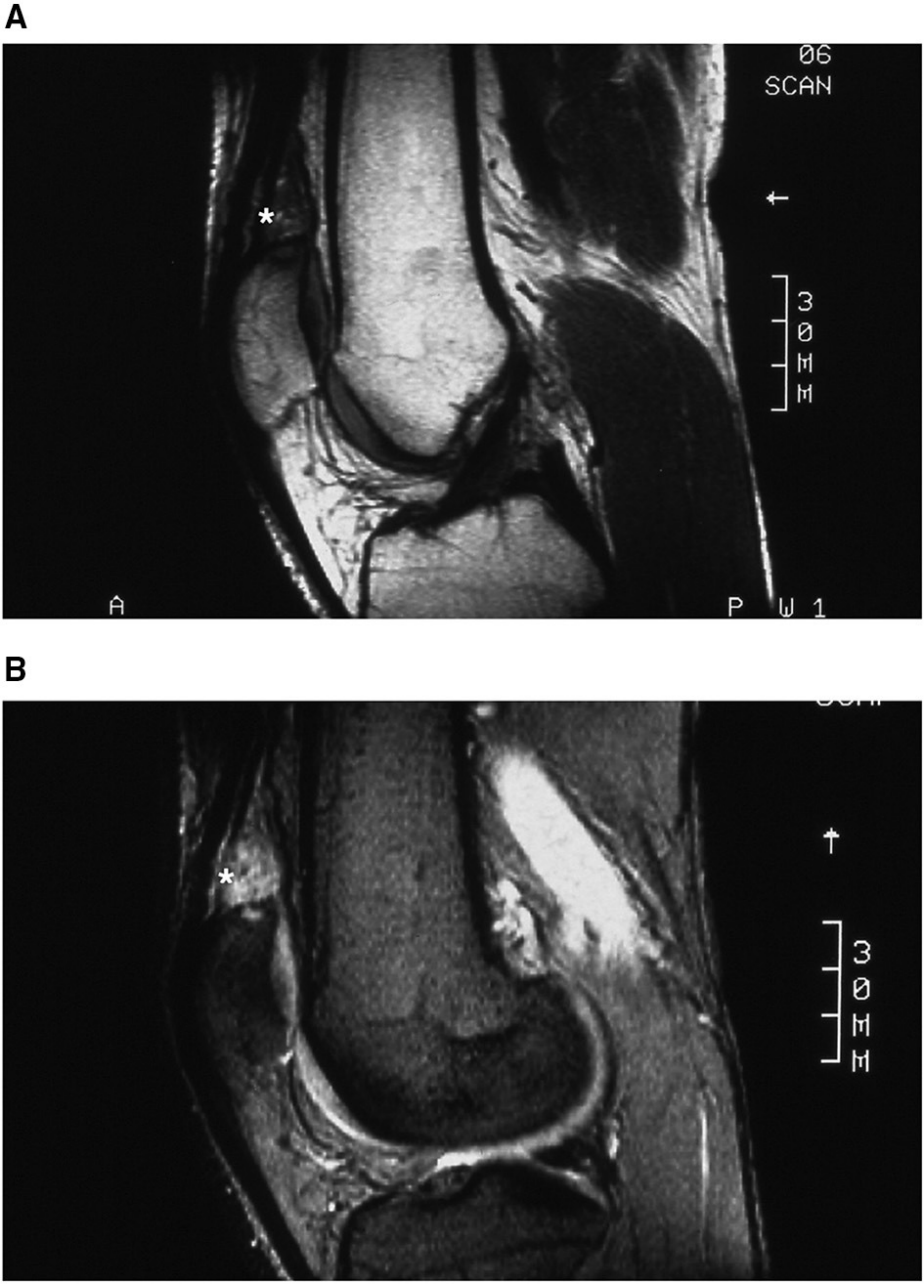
Case 2: suprapatellar SH MRI. Isointense T1 (A) and hyperintense T2 (B) sagittal MR images showing a synovial tumor just over the proximal pole of the patella with intra-osseous invasion of the upper pole of the patella. *Location of the Synovial Haemangioma tumor in the knee.
